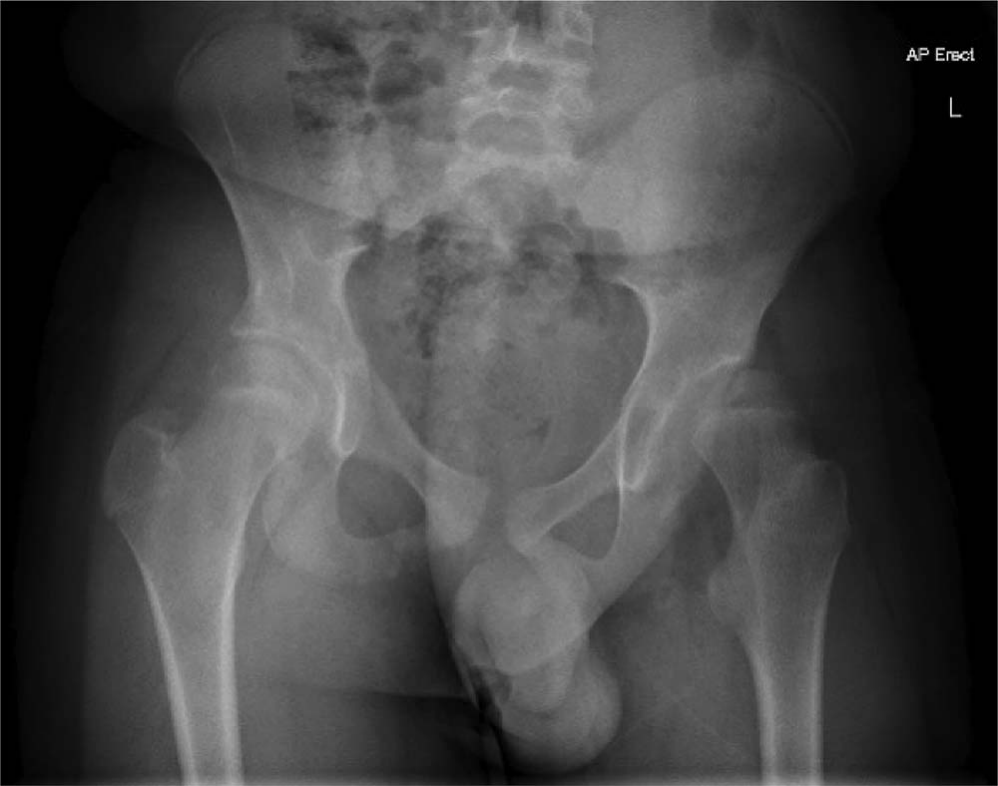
Case 1 pre-operative radiograph.
